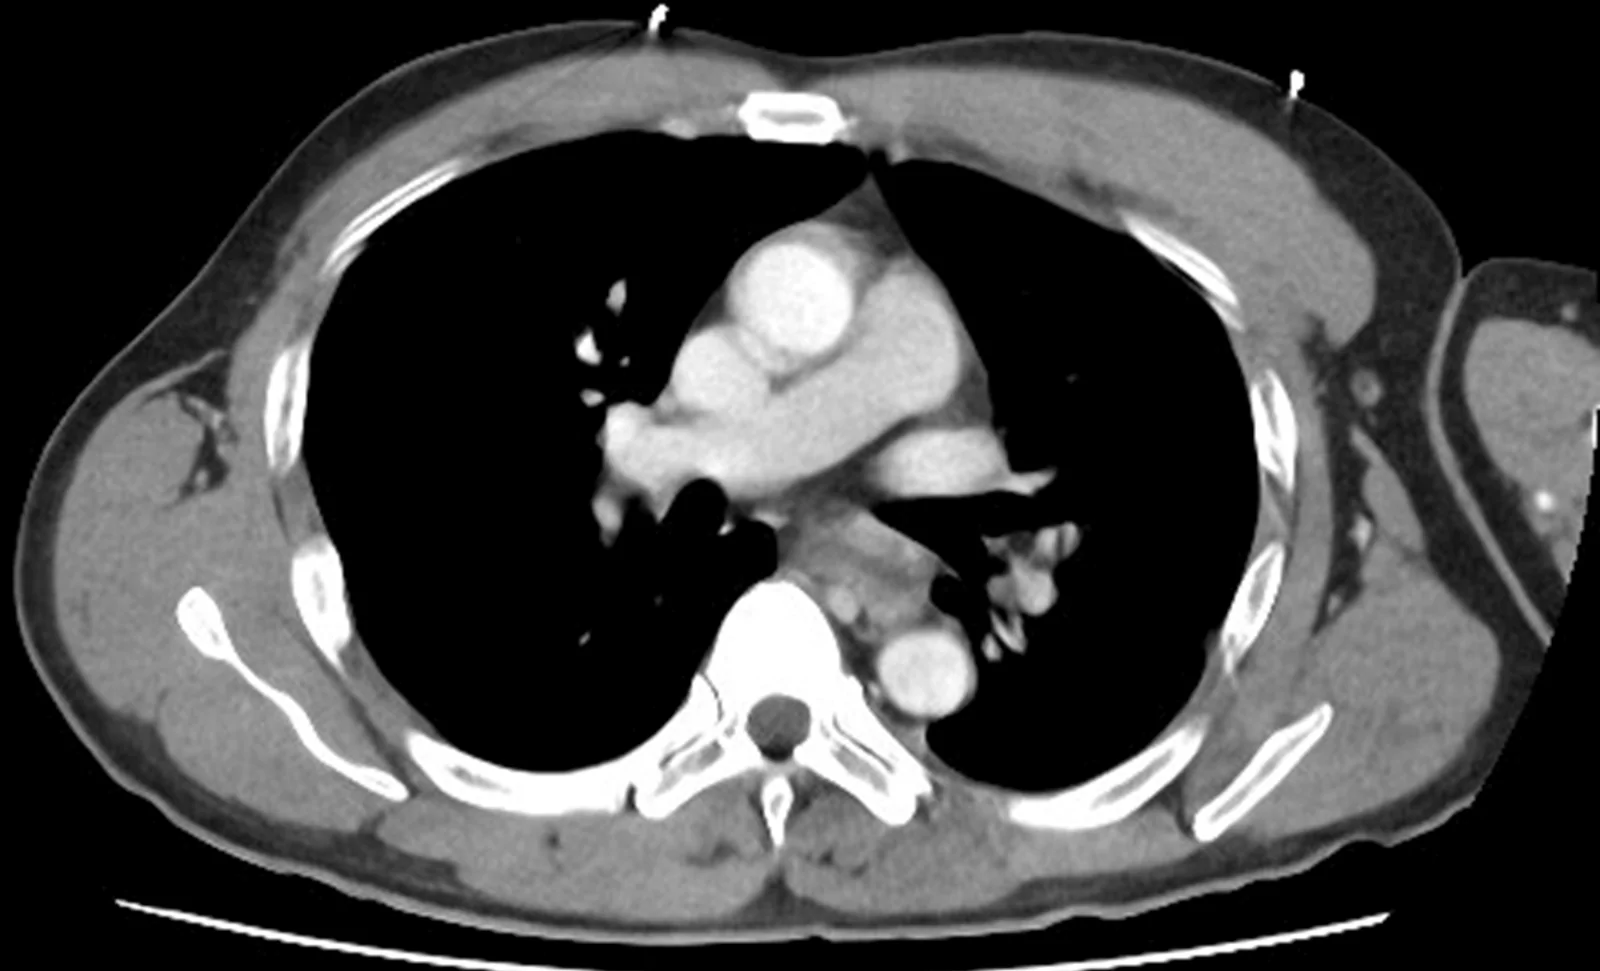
Contrast-enhanced axial CT image of the chest Representative contrast-enhanced axial CT image of the chest obtained during evaluation for occult causes of severe lactic acidosis. No acute thoracic abnormality or infective focus was identified on CT imaging.
